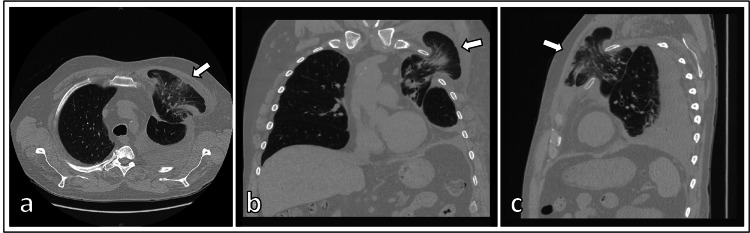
Computed tomography images The images are showing the lung hernia (arrow). a. transversal view, b. coronary view and c. sagittal view.
